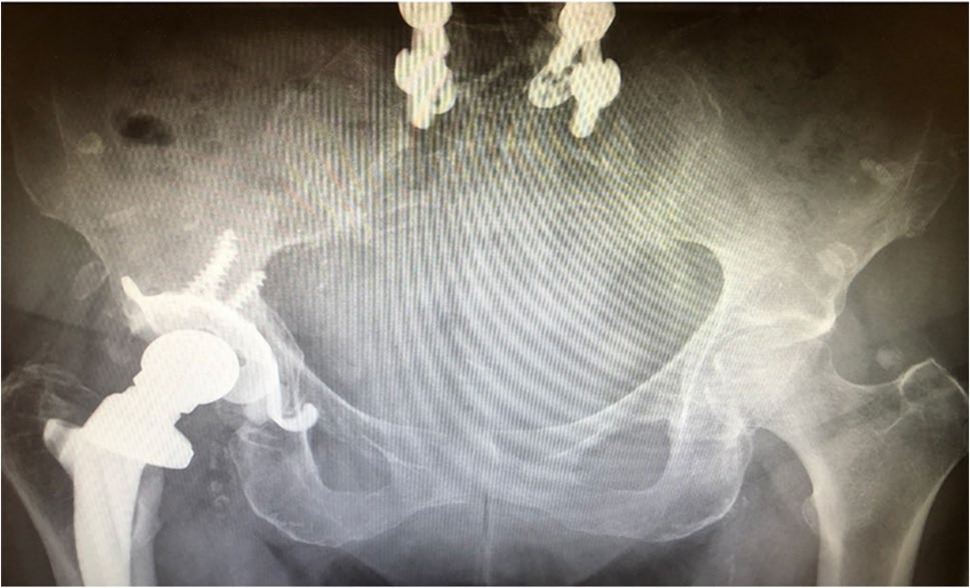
AP pelvis radiograph showing previous right THA, the arthritic changes in the left hip and spinal fusion metalwork
